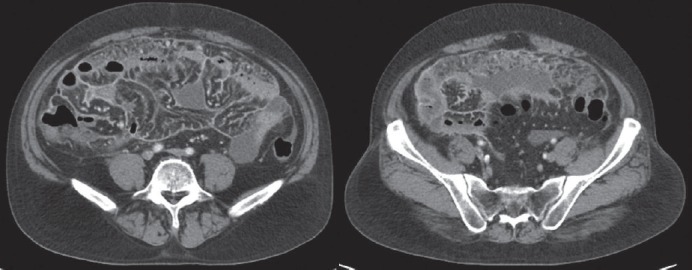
CT scan showing extensive dirty thickening of the peritoneum, fatty stranding and ascites accumulation in the abdomen cavity.
